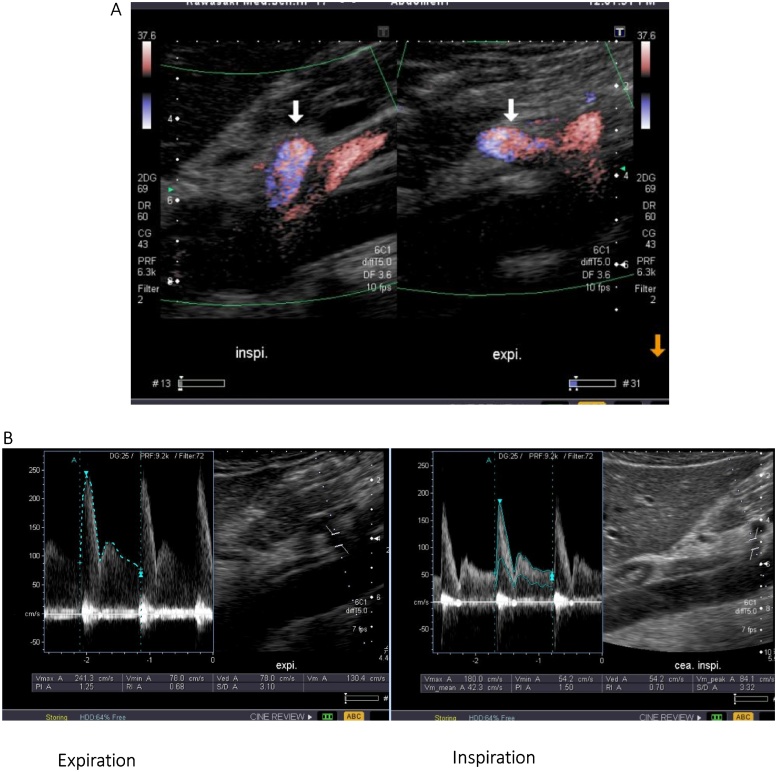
(A) Abdominal doppler echogram showed translocation and deformity of celiac artery between inspiration and expiration(arrow). (B) Abdominal doppler echogram showed the blood flow of celiac artery between inspiration and expiration.
